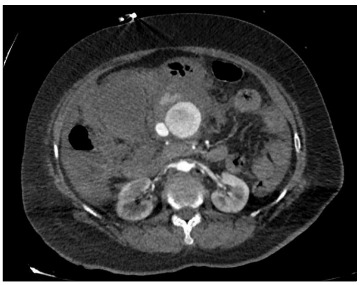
Preoperative late arterial phase computed tomography scan (axial) showing extravasation of intravenous contrast agent.
